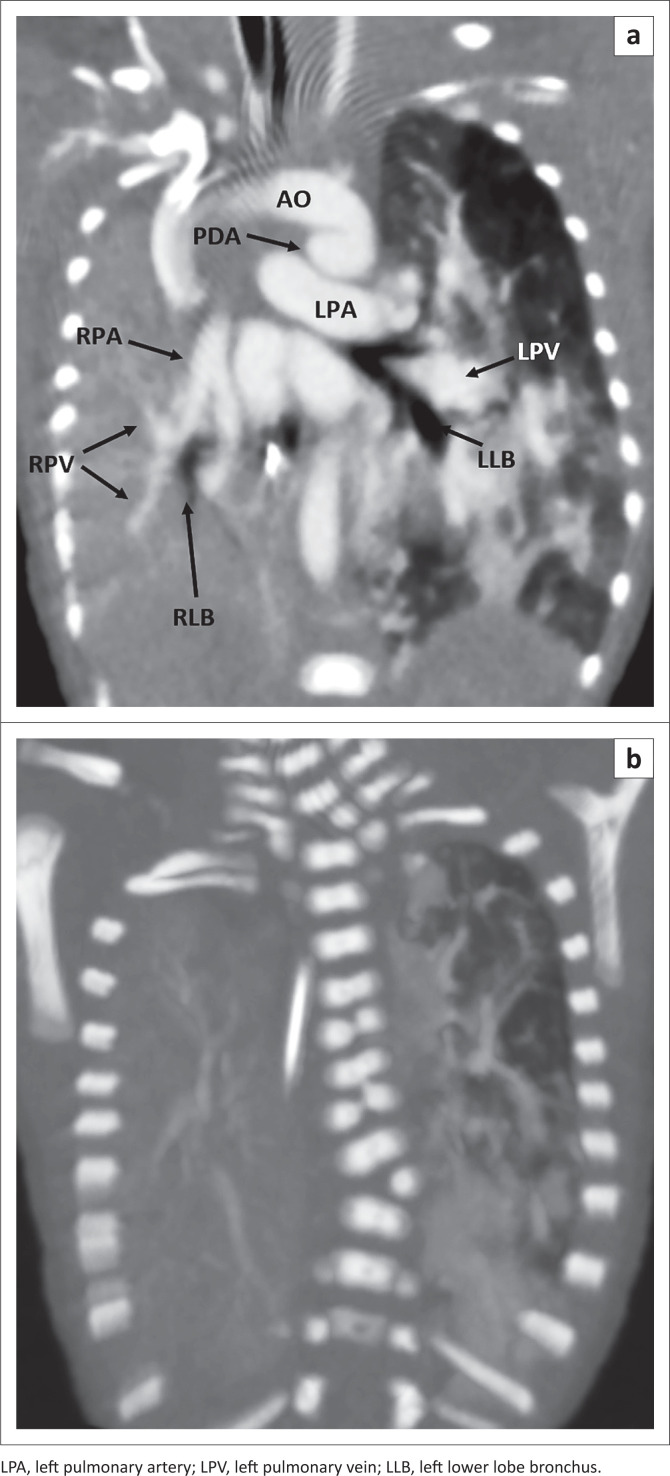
Computed tomography angiography thorax (A) demonstrates hypoplastic right lung, right lower lobe bronchi (RLB), right pulmonary artery (RPA) and veins (RPV). The right hemithorax volume is reduced with ipsilateral mediastinal shift. Large patent ductus arteriosus (PDA) connecting with the aorta (AO). Posterior CT imaging (B) demonstrates left hemivertebrae at C7/T1 and T8/T9, butterfly vertebrae of T5 and T7, and left C7/T1 cervical rib.
